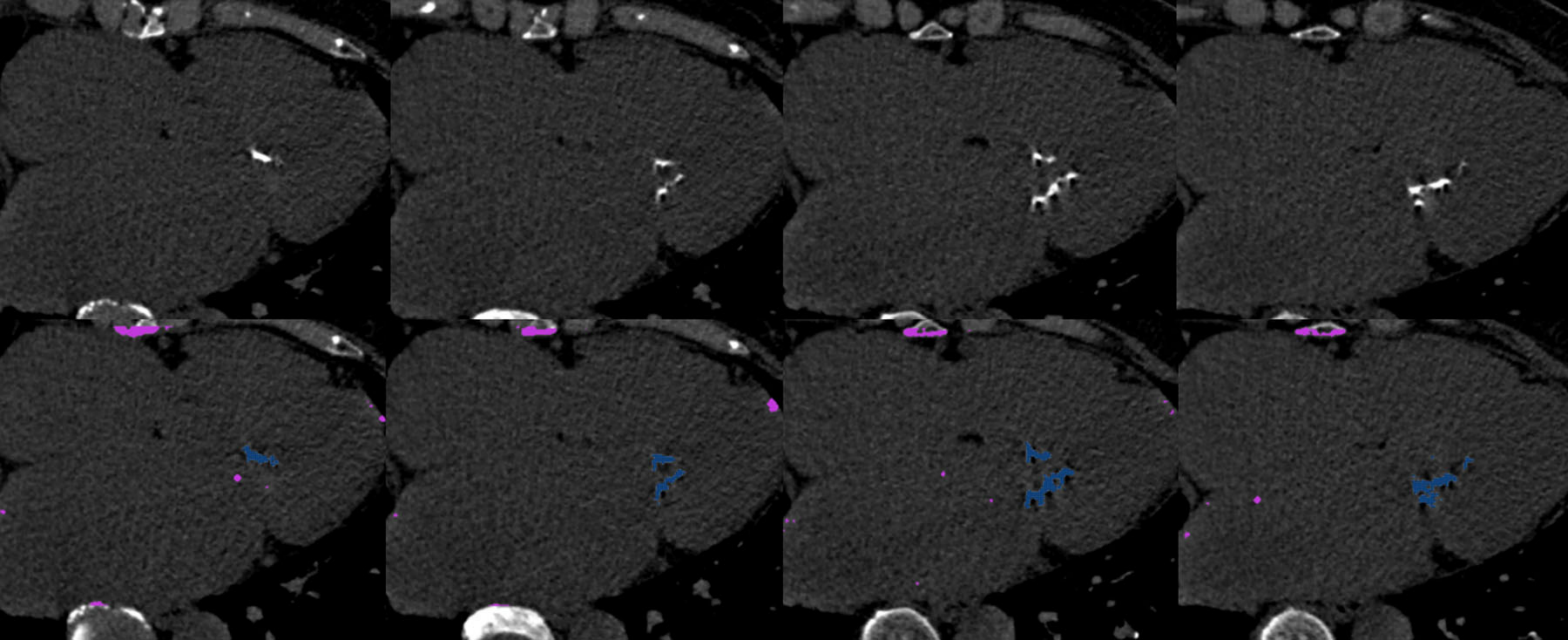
Multiplanar measurement of MAC. Figure 1 showed the case of a mitral valve disease with MAC. MAC score was evaluated by CT. MAC volume was measured at 1372 mm^3^, MAC score was measured at 1767. CT, computed tomography; MAC, mitral valve calcification.
